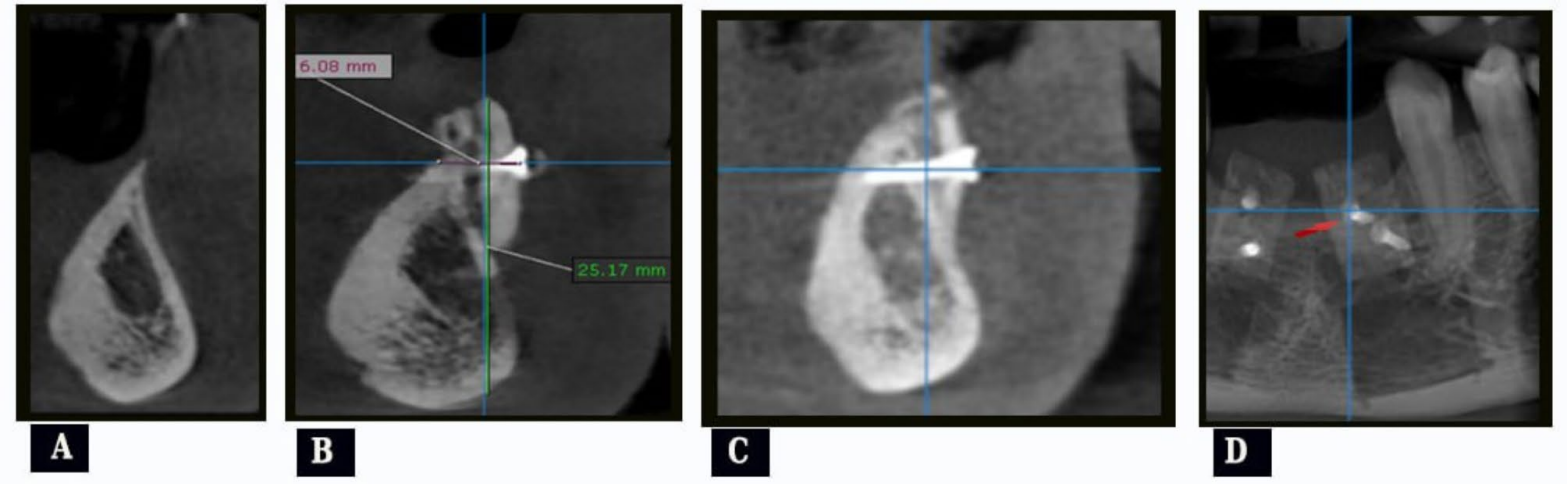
(case#3): A, Preoperative CBCT cross sectional view showing atrophied alveolar ridge; B, Immediate postoperative CBCT cross sectional view showing measurement of alveolar ridge height and width; C, CBCT cross sectional view showing alveolar ridge 6 months after grafting; D, Adaptation of the two reference lines to be crossed at the head of the coronal screw in CBCT panoramic view (arrow)
